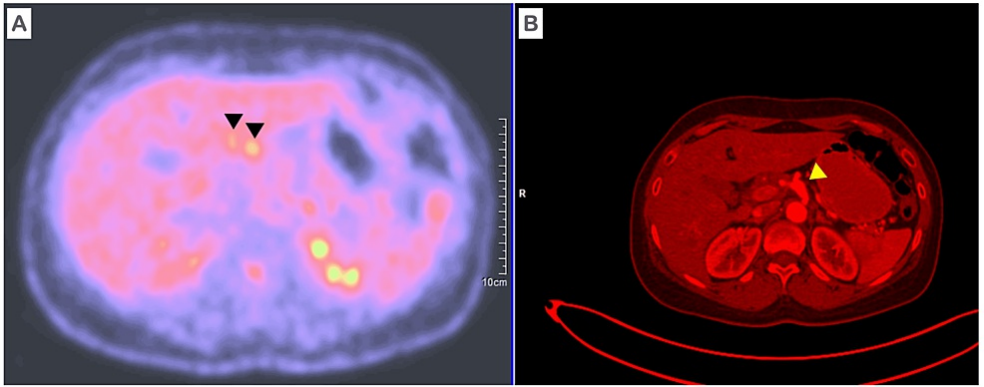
Abdominal 18-fluorodeoxyglucose positron emission tomography (FDG-PET) scan Metabolic hyperactivity in the hepatic artery A. Black head arrows B. Yellow head arrow.
